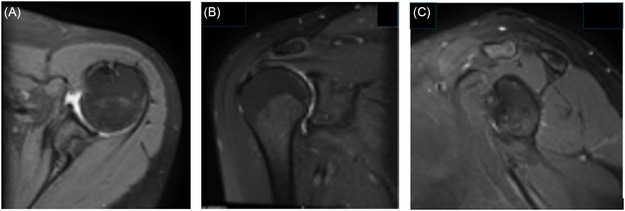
Preoperative magnetic resonance images (A) Left shoulder, axial magnetic resonance image with posteriorly subluxated humeral head and subscapularis deficiency. (B) Left shoulder, coronal magnetic resonance image with intact supraspinatus tendon. (C) Left shoulder, sagittal magnetic resonance image showing anchors from prior open Bankart repair and capsulorrhaphy.
